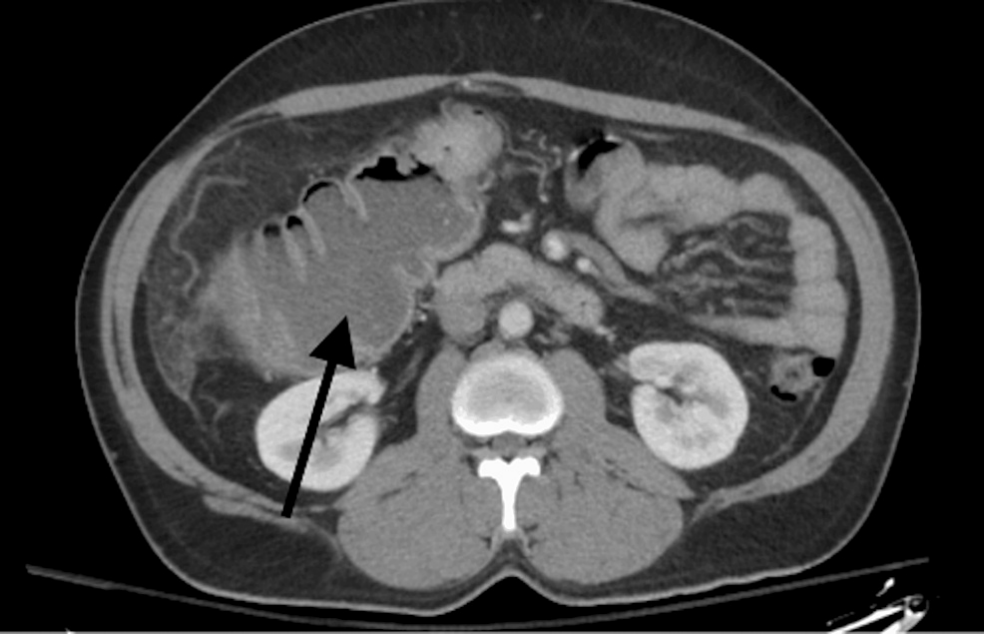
CT abdomen and pelvis with contrast, axial view, demonstrating large bowel obstruction in the transverse colon.
